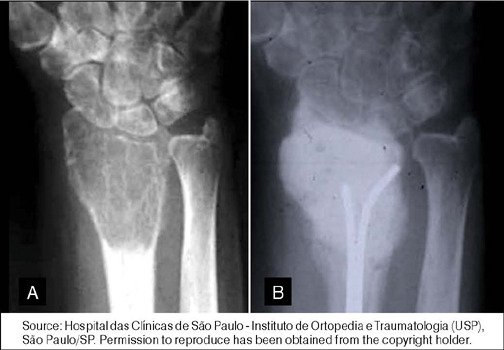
(A) X-ray of the wrist showing a Campanacci grade 3 giant cell tumor of bone (GCTB) of the distal radius; (B) The patient was treated with intralesional curettage, adjuvants, and cement filling.
